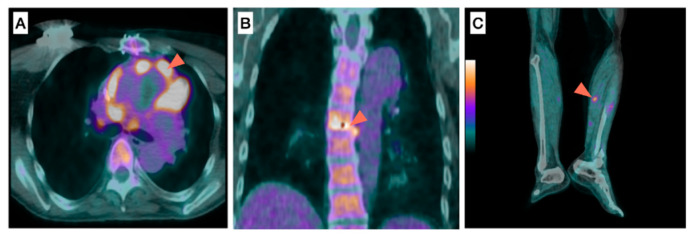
Role of ^18^F-FDG-PET/CT for the detection of septic emboli in a patient with infective endocarditis. (A) prosthetic valve, (B) spine, (C) anterior tibial artery.
